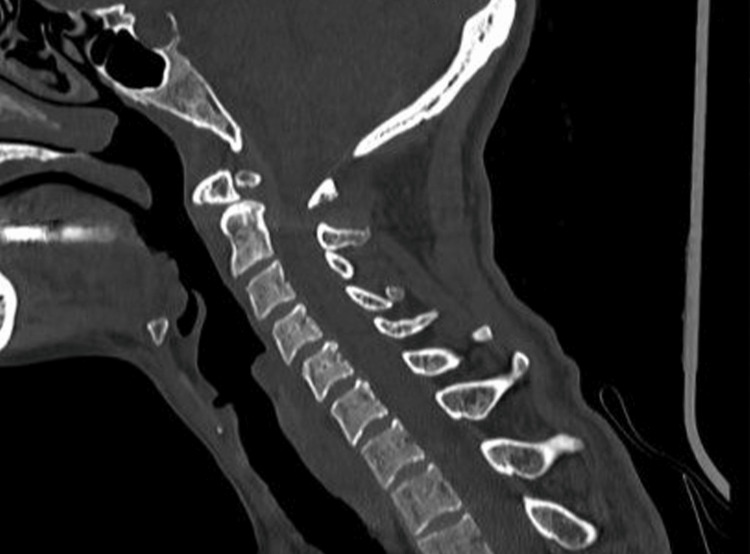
Computed tomography (CT) demonstrated straightening of the examined cervical spine, reflecting paravertebral muscle spasm.
